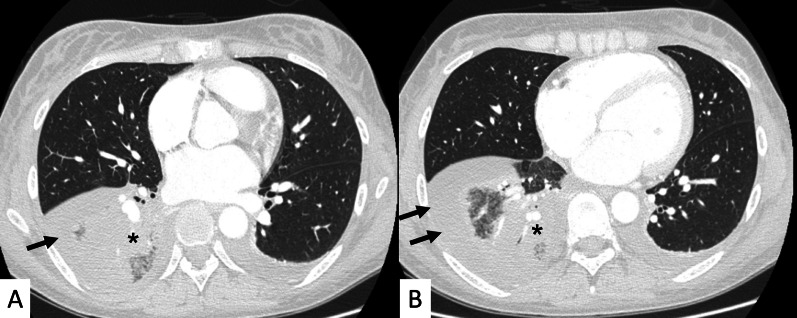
A 37-year-old patient with ALP, characterized by dyspnea, fever and pleuritic pain; on HRCT images (A, B), bilateral pleural effusions (black arrows) and right lower lobe consolidation (black asterisk) are clearly recognizable
